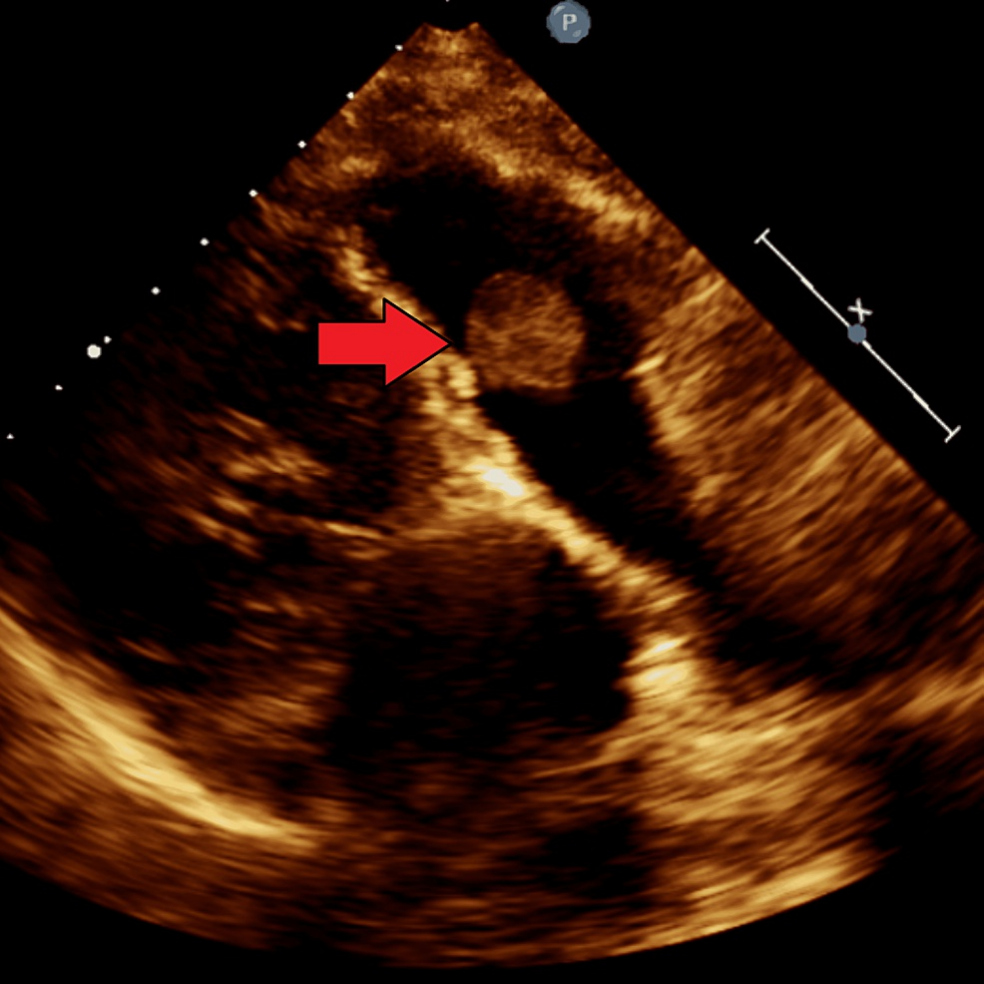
A well-circumscribed and homogenous mass can be seen on transthoracic echocardiogram.
